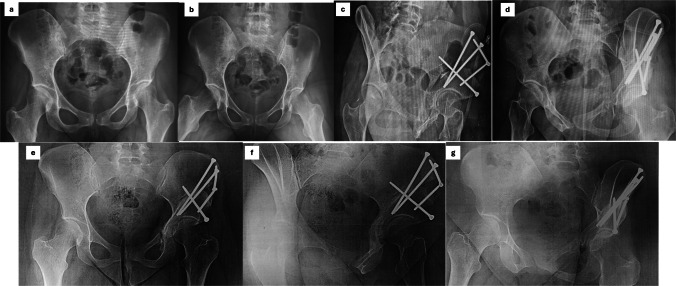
A 41-year-old female with symptomatic adult acetabular dysplasia, predominantly affecting the left hip, treated with PAO. a, b Preoperative radiographs demonstrating acetabular dysplasia with reduced LCEA and increased AI. c, d Immediate postoperative radiographs showing adequate acetabular reorientation with correction of both LCEA and AI, stabilized using five 4.5-mm cortical screws. e–g Final follow-up radiographs demonstrating maintained acetabular correction, complete union
